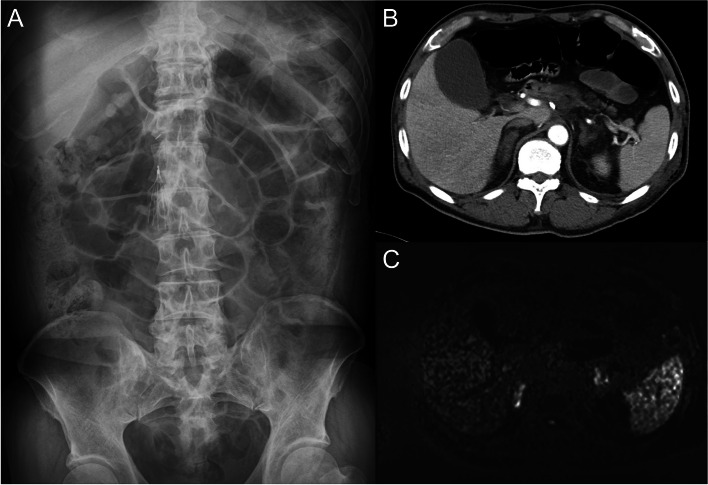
Postoperative findings. A Abdominal radiography shows intestinal gas. B Contrast-enhanced computed tomography reveals adrenal swelling and increased surrounding retroperitoneal adipose tissue density. C Abdominal magnetic resonance imaging reveals high-intensity areas in both adrenal glands on diffusion-weighted images
